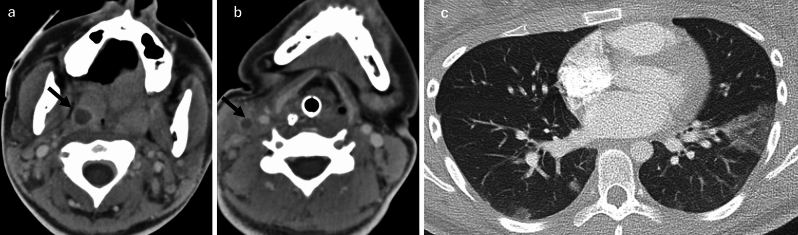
Septic embolism with Lemierre’s syndrome. A woman in her 20 s was diagnosed with a septic embolism secondary to Lemierre’s syndrome. Contrast-enhanced CT of the neck revealed an abscess in the right palatine tonsil (a) and thrombosis of the right internal jugular vein (b). Chest CT demonstrated a triangular, wedge-shaped ground-glass opacity with well-defined margins abutting the pleura in the left lower lobe; these findings are consistent with pulmonary infarction. The periphery of the opacity was denser than the center. Multiple peripheral nodules were also noted in the right lower lobe (c)
